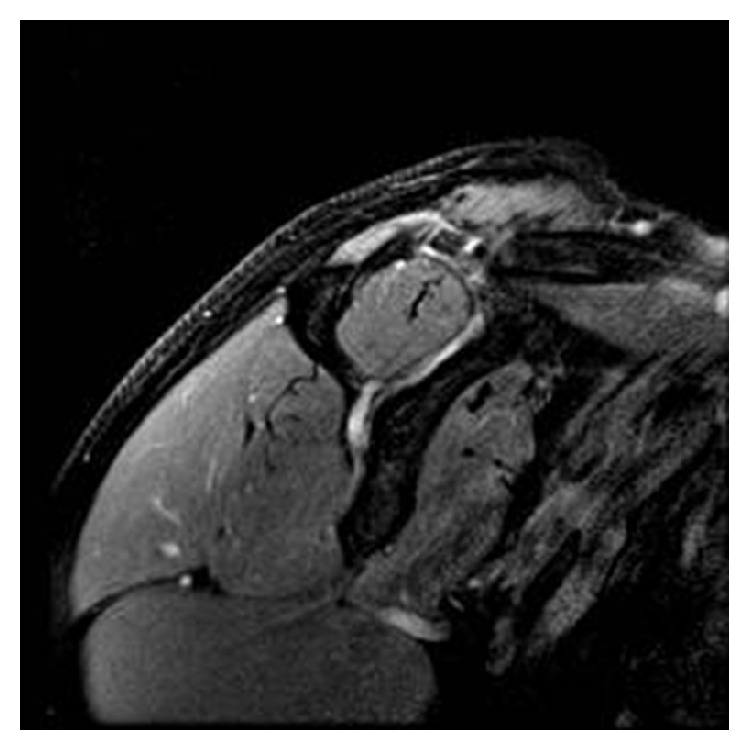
Magnetic resonance imaging of the acromioclavicular joint in DP fat-suppression sequence demonstrates the osteolysis with no soft tissue reaction or bone edema.
